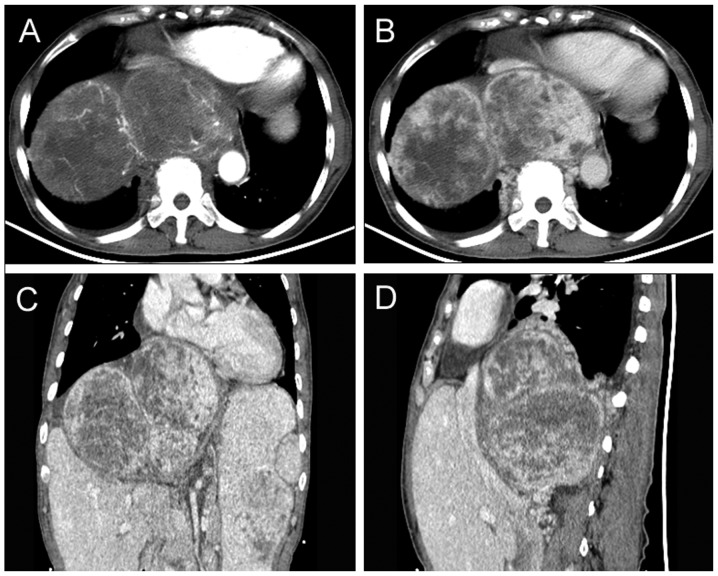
(A) Arterial-phase CT scan. The tumor shows moderate to marked enhancement, with the tumor edge visible for tortuous vascular shadow. (B) Venous-phase CT scan demonstrating sustained enhancement of the tumor. Nonenhanced areas suggest central cystic degeneration and necrosis. (C) Coronal and (D) sagittal reconstructed images: The tumor is lobulated, comprised of three masses. The heart, liver and right kidney were pressured significantly due to the tumor mass effect. CT, computed tomography.
